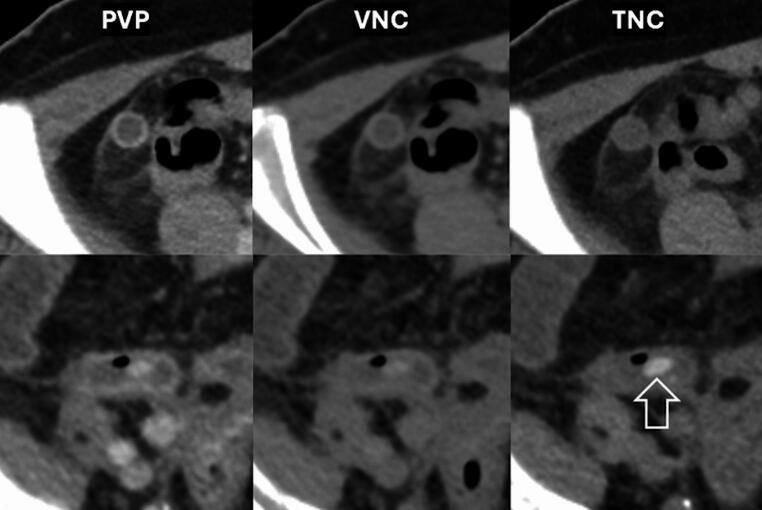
Example of cases with clear absence (top row) and presence (bottom row) of appendicoliths on portovenous phase (PVP), virtual noncontrast (VNC), and true noncontrast (TNC) dual-energy CT. Arrow = appendicolith
